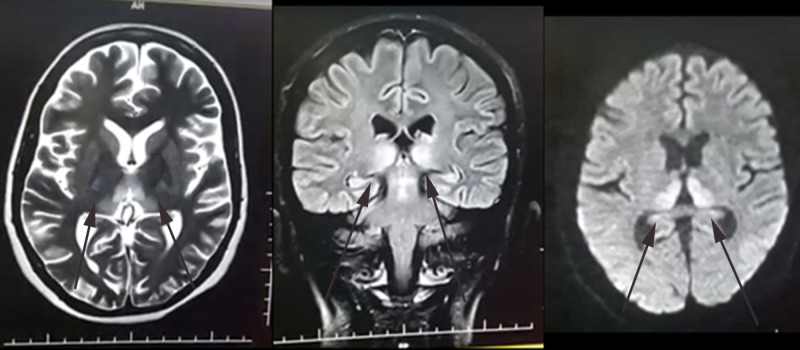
Magnetic resonance imaging (MRI) of the brain showing hyper-intense and diffusion restricted areas in the peri-tectal region and bilateral medial thalami
